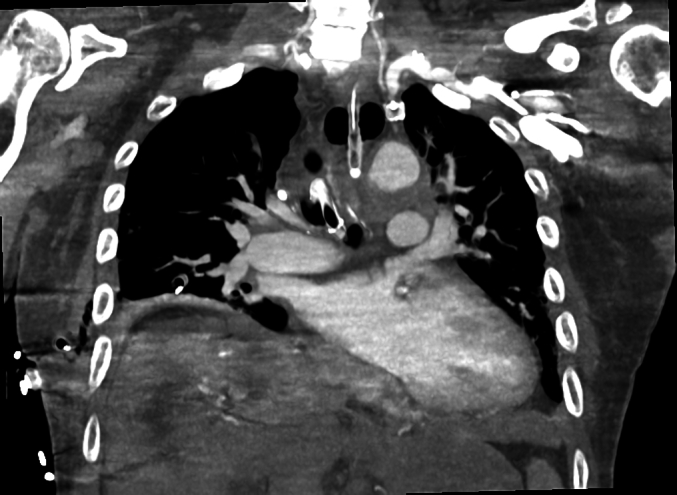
Coronal section of CT chest performed with IDC in situ, occluding the fistula.
